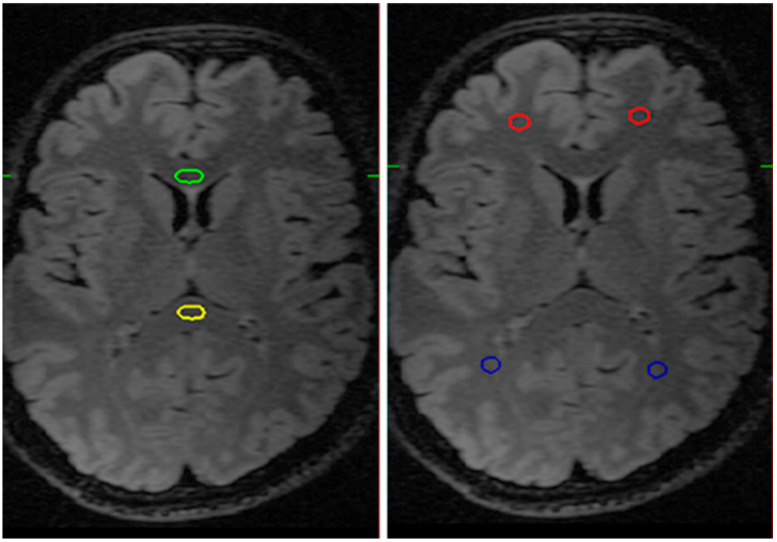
Representative placement of measurement regions on axial FLAIR images. The genu of the corpus callosum is shown in green, the splenium in yellow, bilateral frontal WM in red, and bilateral posterior WM in blue. Right- and left-sided frontal and posterior WM measurements were averaged to generate one frontal WM value and one posterior WM value for each participant. These regions were used for the extraction of ADC, D, D*, and f values. ADC = apparent diffusion coefficient; D = true diffusion coefficient; D* = pseudo-diffusion coefficient; f = perfusion fraction; ROI = region of interest; WM = white matter.
